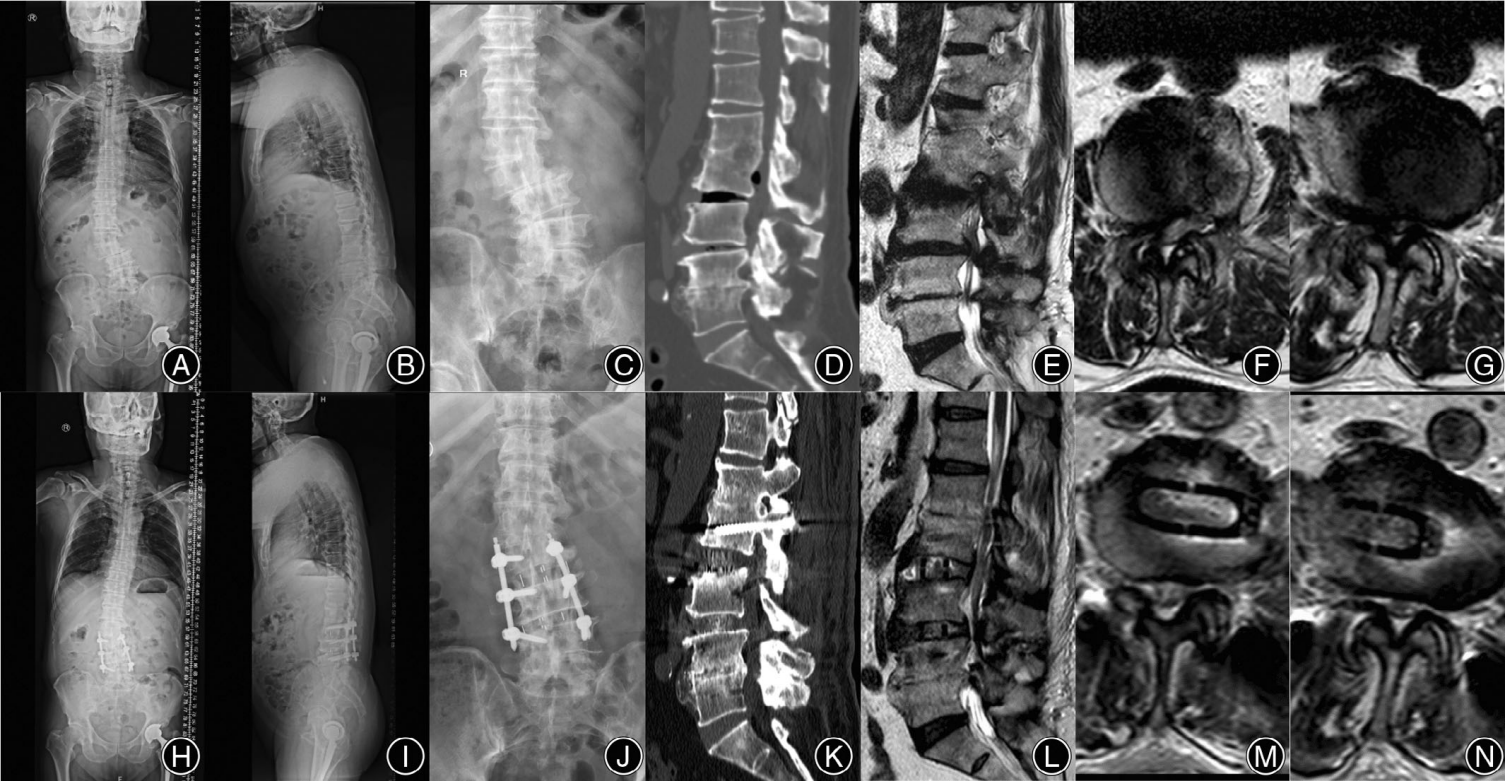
Whole spine X‐ray showed significant L2 lateral slip, reduced lumbar lordosis, and sagittal imbalance (A–C). CT showed spontaneous fusion of L_1/2_ and L_4/5_ interbody spaces and an intradiscal vacuum phenomenon of L_2/3_ and L_3/4_ (D). MRI showed severe spinal stenosis at L_2/3_ and L_3/4_ segments, and the Schizas grade was C and D, respectively (E–G). Postoperative X‐rays showed that the lateral slip of L_2_ and L_3_ vertebras was significantly improved, and CT showed that the height of L_2/3_ and L_3/4_ disc space increased. MRI showed that the spinal canal stenosis degree of L_2/3_ and L_3/4_ were both improved to Schizas grade B (L–N).
